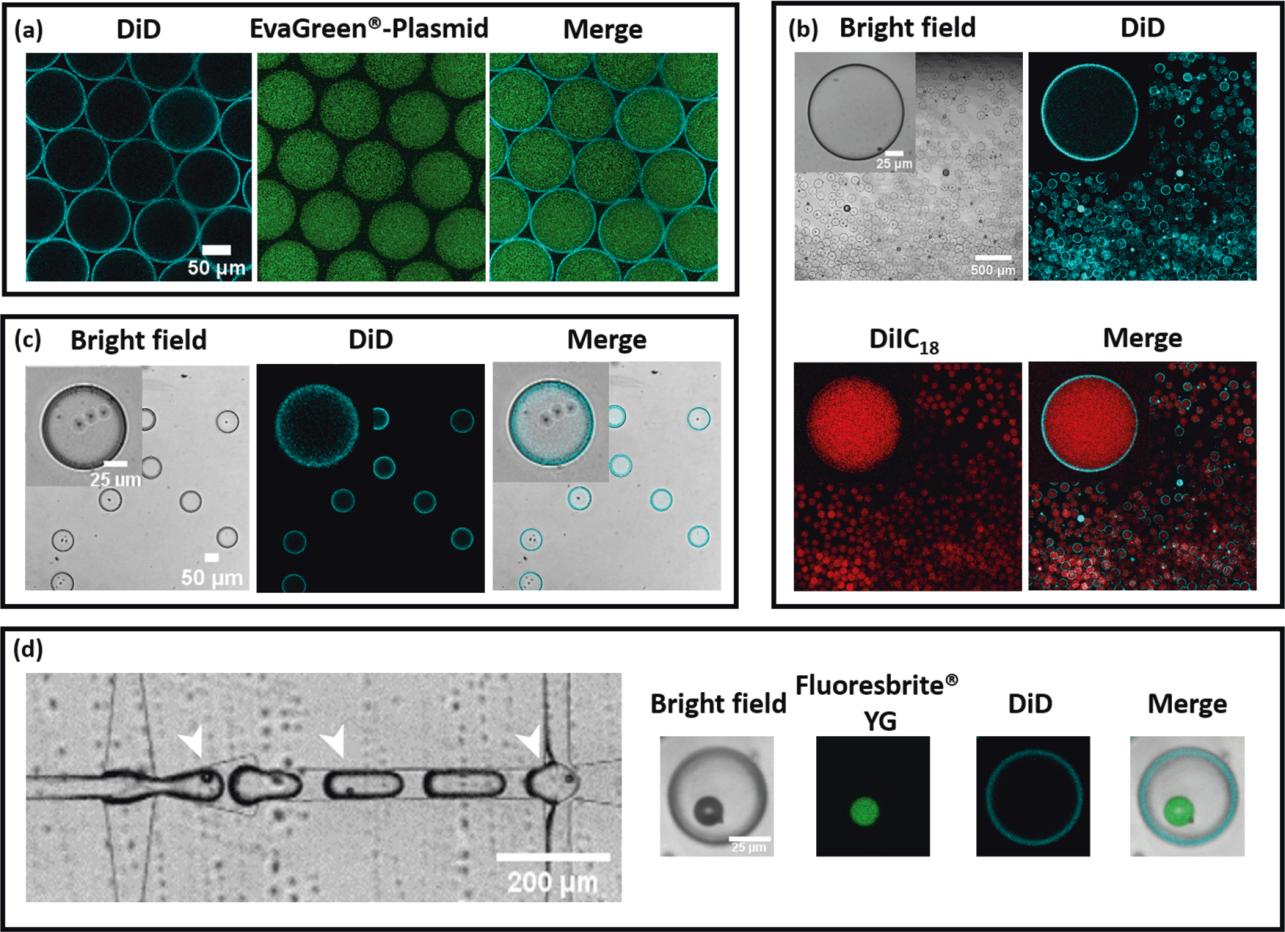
Microfluidic production of artificial cells. a Encapsulating EvaGreen^®^-plasmid DNA, b showing compartmentalization with SUVs, c encapsulating fibroblast cells along with the culture media, and d encapsulating microplastics such as styrene microspheres.
